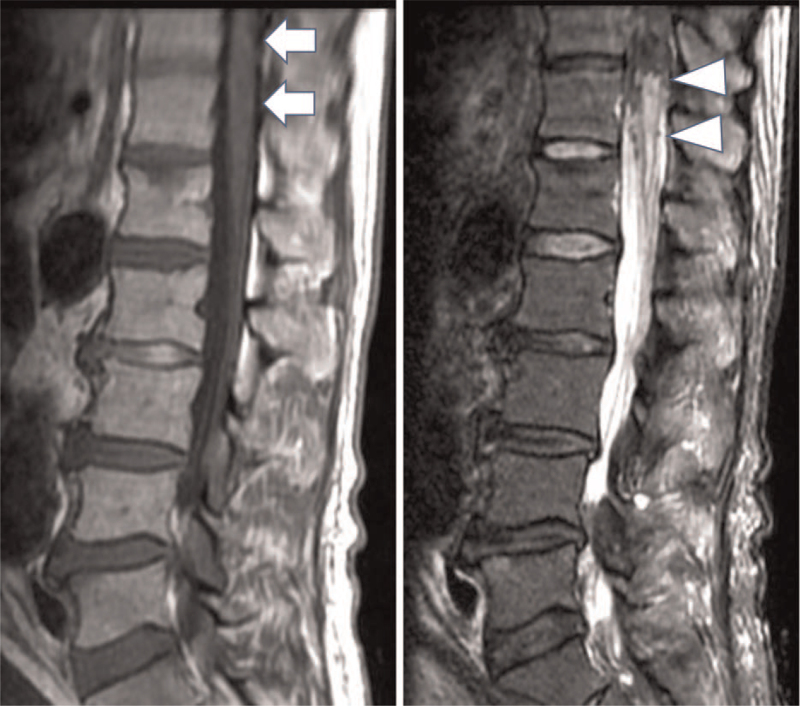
Spinal cord infarction is identified on MRI. (A) T1WI (arrow). (B) T2WI (arrow head). MRI = magnetic resonance imaging, WI = weighted image.
